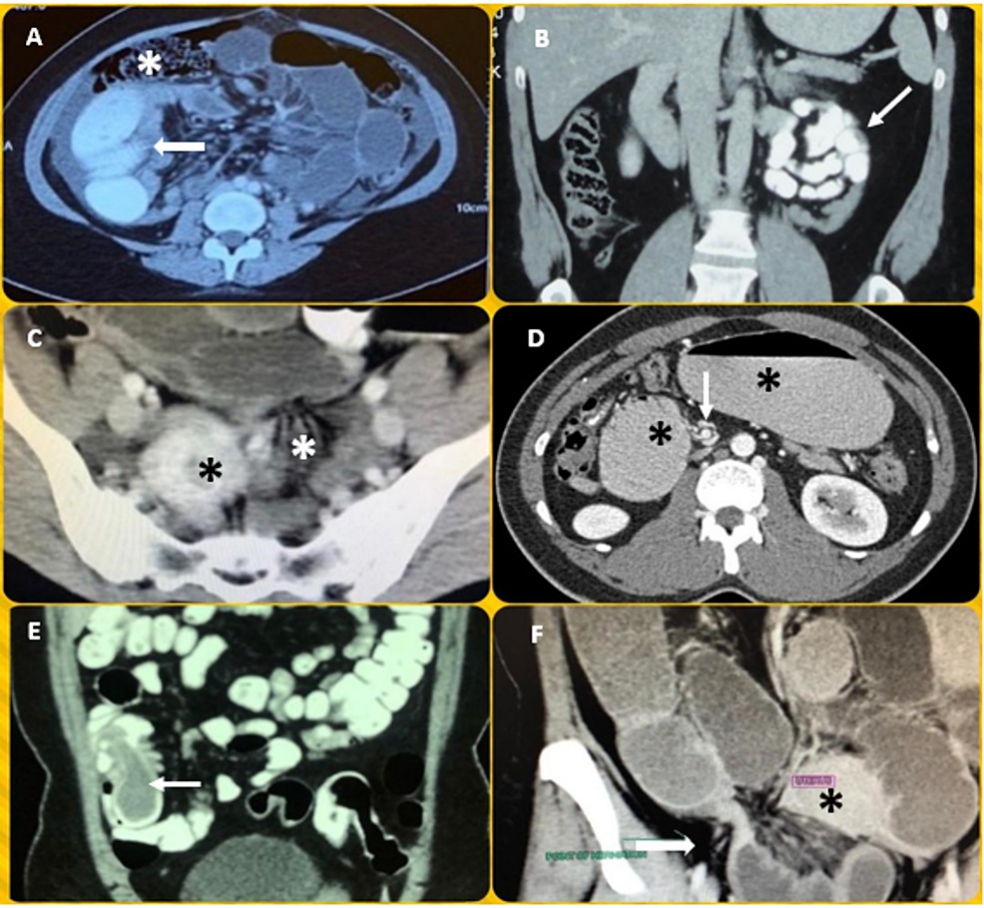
CECT of the abdomen appearances of some of the rare and exciting cases (A) Axial view of right PDH showing encapsulated contrast-filled dilated ileal loops (white arrow) posterior to ascending colon (white asterisk); (B) coronal view of left PDH showing a cluster of encapsulated contrast-filled ileal loops to the left of midline; (C) coronal view of left BLH showing herniated ileal loops through the broad ligament defect (white asterisk) pushing the uterus (black asterisk) a little to the right; (D) axial view of midgut malrotation showing the dilated stomach and duodenum (black asterisks) and the “whirlpool” sign of mesenteric vessels (white arrow); (E) coronal view of ileo-ileal intussusception revealing “sausage” sign (white arrow); and (F) coronal view of postoperative adhesion-related right-sided internal hernia showing point of transition (white arrow) on the right lateral side of the uterus (black asterisk). CECT, Contrast-enhanced computed tomography; PDH, paraduodenal hernia; BLH, broad ligament herniation.
